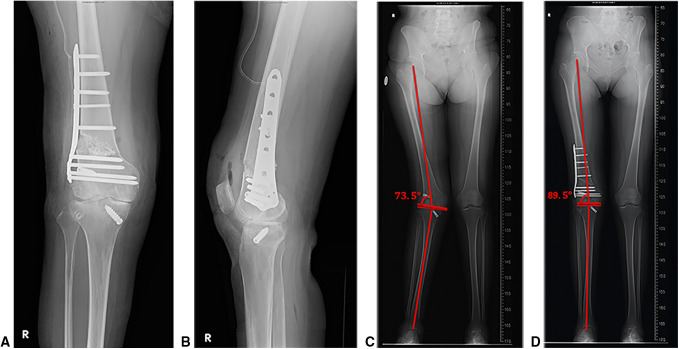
Postoperative knee radiograph (A, B) and a comparison of the lower limb scanogram (C, D).
